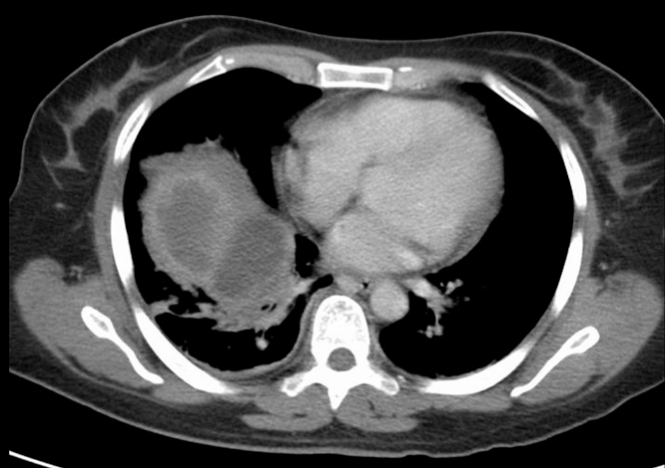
Initial abdominal CT scan demonstrating hepatic abscess.
